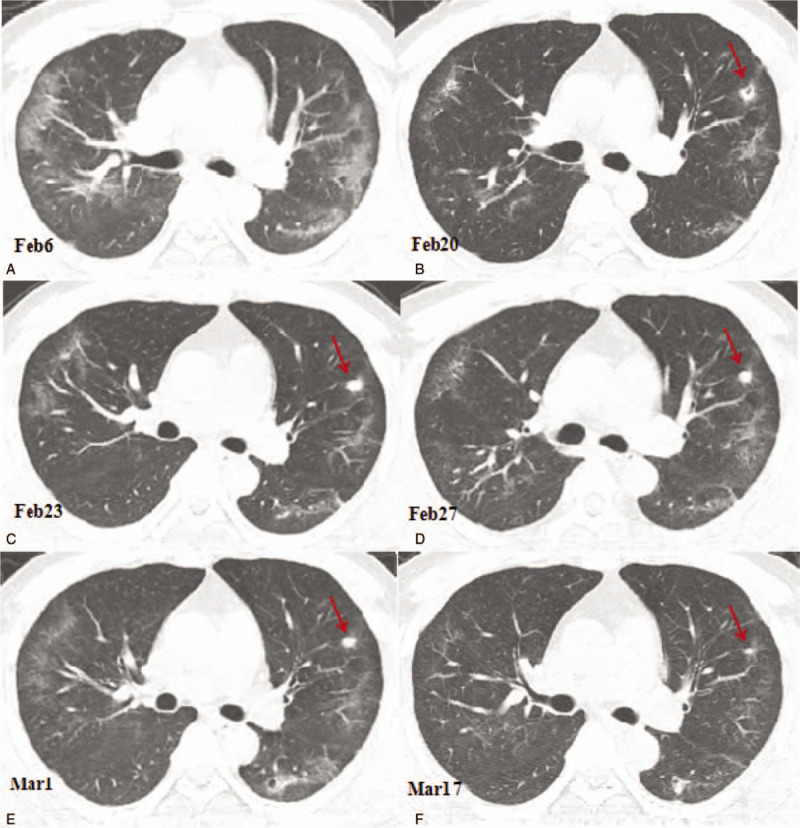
Computed tomography (CT) images showing the changes of the cavity in the anterior segment of the left upper lobe. (A) A CT image obtained on February 6, 2020 showing the ground glass opacities and linear opacities in the lungs predominantly distributed in the peripheral third of the lungs. (B) A CT image obtained on February 20, 2020 showing the reduced ground glass opacities area. A small cavity was found in the anterior segment of the left upper lobe with a size of 9.3 × 6.7 mm. (C) A CT image obtained on February 23, 2020 showing consolidation of the small cavity in the anterior segment of the left lobe with a size of 7.8 × 6.3 mm (arrow); (D) A CT image obtained on February 27 showing consolidation of the cavity in the upper segment of the left upper lobe with the size slightly reduced to 6.5 × 5.5 mm (arrow); (E) A CT image obtained on March 1, 2020 showing the reduced cavity in the anterior segment of the left upper lobe, with a size of 5.0 × 4.5 mm (arrow). (F) A CT image obtained on March 17, 2020 showing that the ground glass opacities in the lungs were almost completely absorbed. The solid nodule in the anterior segment of the left upper lobe had significantly reduced in size, with a size of 2.5 × 1.5 mm (arrow).
